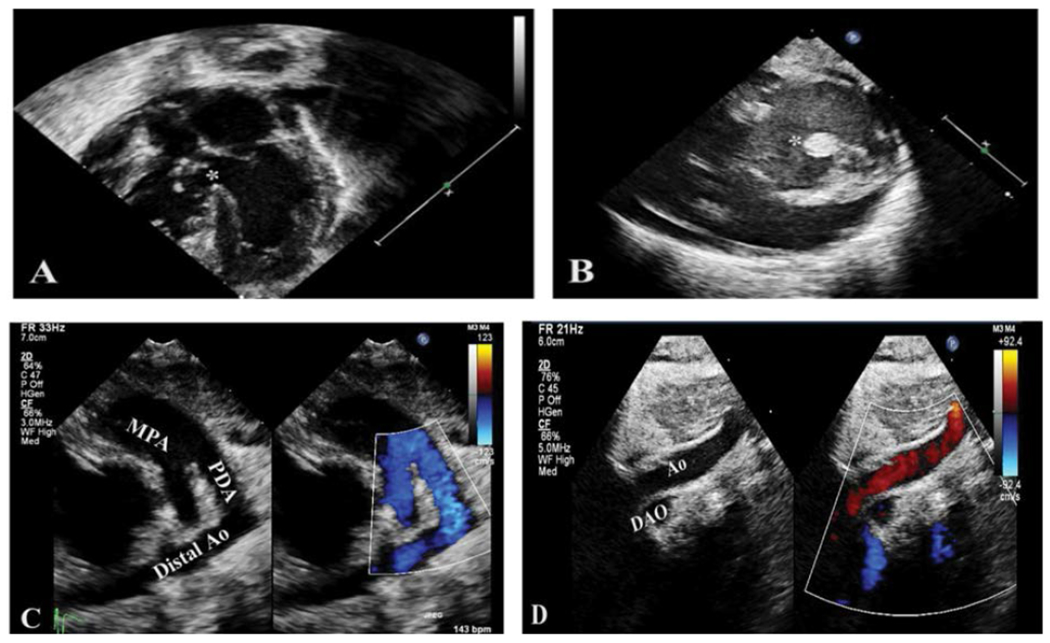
Representative echocardiography images of proband showing multiple cardiac malformations. (A) 5-chamber view showing VSD and posterior malaligned conal septum (asterisk); (B) Parasternal short axis view showing VSD (asterisk); (C) Color Doppler interrogation of the ductus arteriosis (PDA) showing origin at the main pulmonary artery (MPA) and ductal arch with unobstructed right to left flow to the distal aorta (Distal Ao). (D) Suprasternal notch view of aortic arch (Ao) demonstrating interruption without communication with the descending aorta (DAO).
